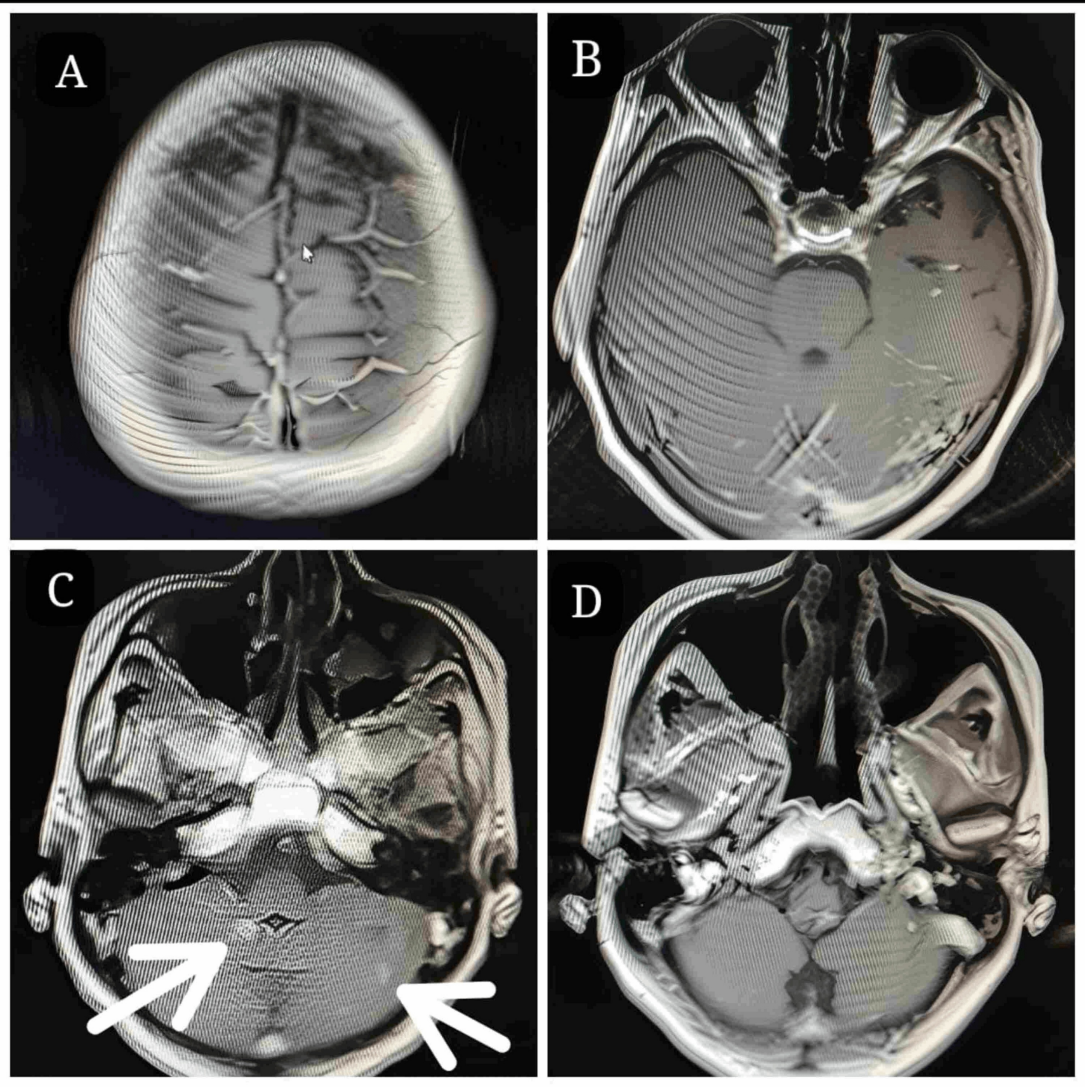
Contrast-enhanced MRI of the brain at the eighth month follow-up with almost complete regression (A, B, and D) of intracranial metastatic deposits of the primary left breast invasive ductal carcinoma and residual deposits (C). (A) Axial section with parts of frontal, parietal, and occipital lobes. (B) Axial sections of the temporal, parietal, and occipital lobes. (C) Axial section of mid-cerebellum with white arrows indicative of residual metastatic deposits. (D) Axial section of the lower cerebellum and brainstem.
